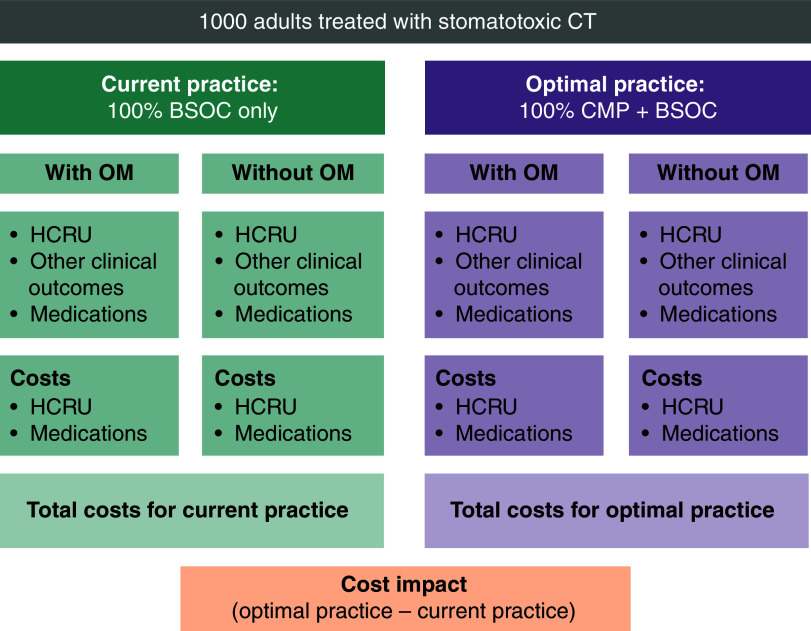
Model structure. BSOC: Best supportive oral care; CMP: Chemo Mouthpiece, CT: Chemotherapy; HCRU: Healthcare resource utilization; OM: Oral mucositis.
